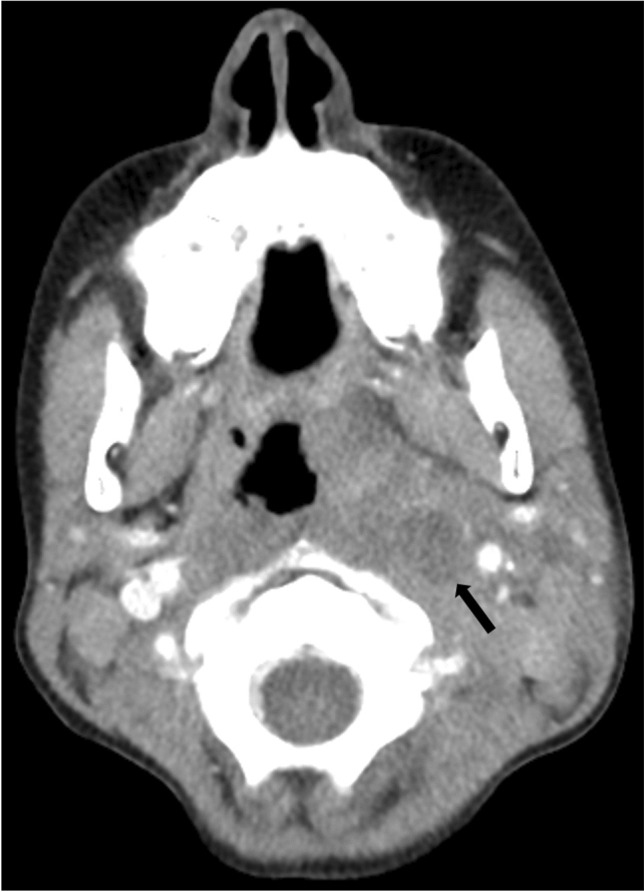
Axial computed tomography image (soft tissue window) without contrast agent administration in a 7-year-old boy with a left retrotonsillar abscess due to tonsillitis (*arrow*)
